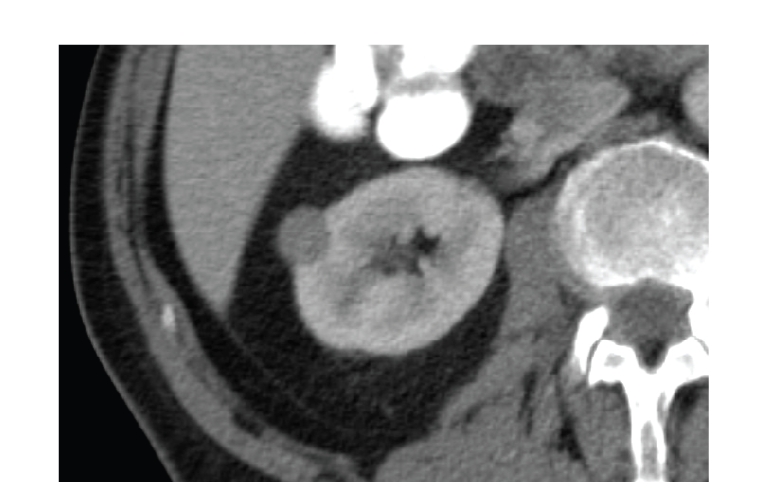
Papillary renal cell carcinoma. Contrast-enhanced CT shows small homogeneous
